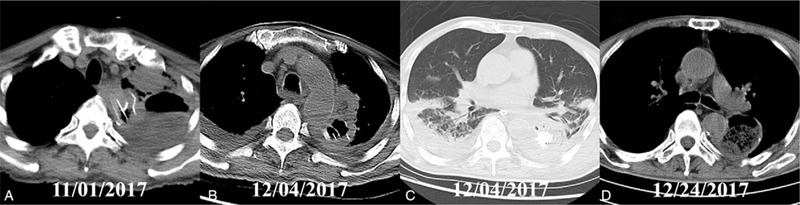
The radiographic images of the patient. (A) The CT on his readmission showed left-sided pleural effusion, in suspicious of late-onset anastomotic leakage. (B) Bilateral aspiration pneumonia was indicated 1 month after the chest tube drainage. (C) Left-sided atelectasis and residual pleural effusion after 1 month of chest tube drainage. (D) The pulmonary field turned to be clean after 20 days of naso-leakage extraluminal drainage.
